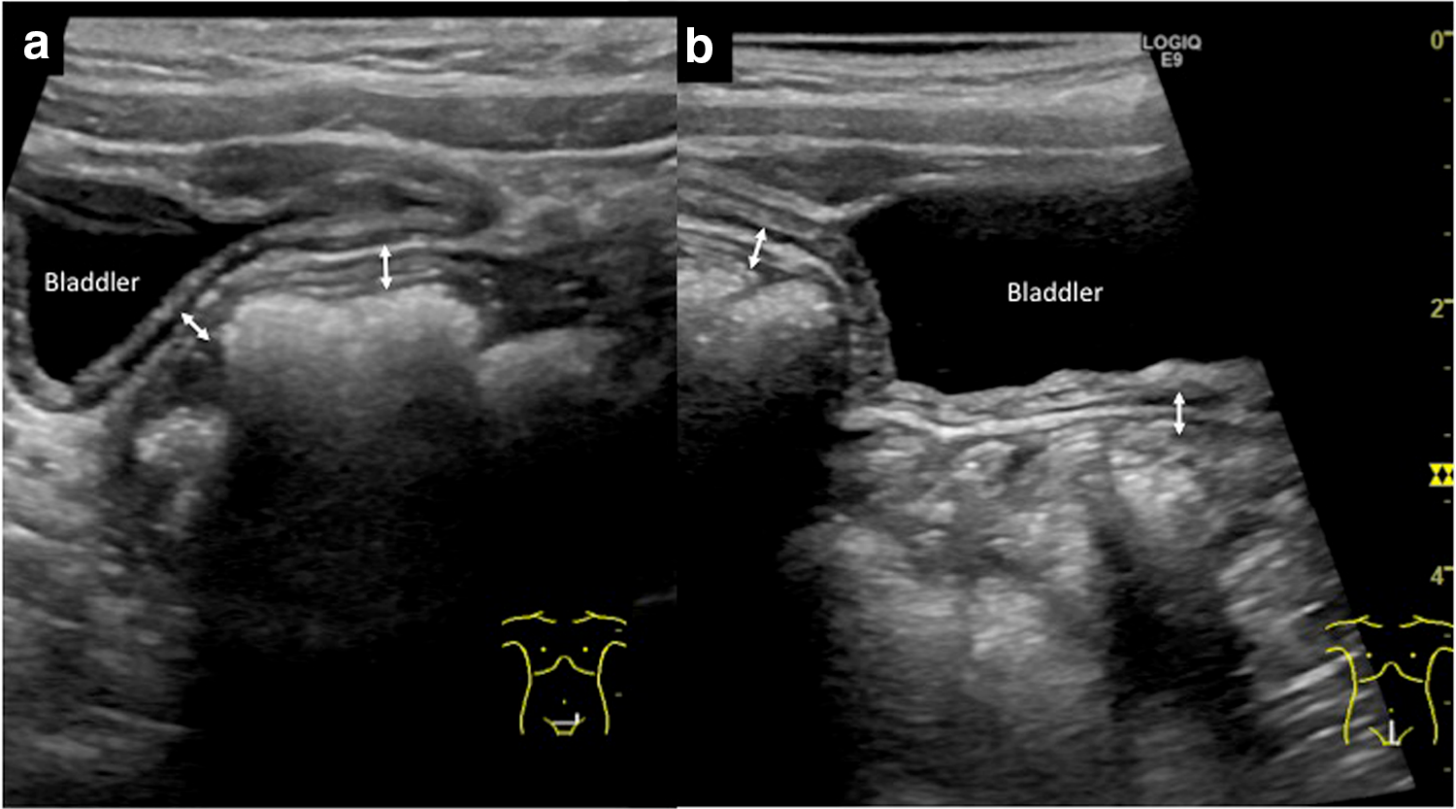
Abdominal ultrasonography at discharge. a
